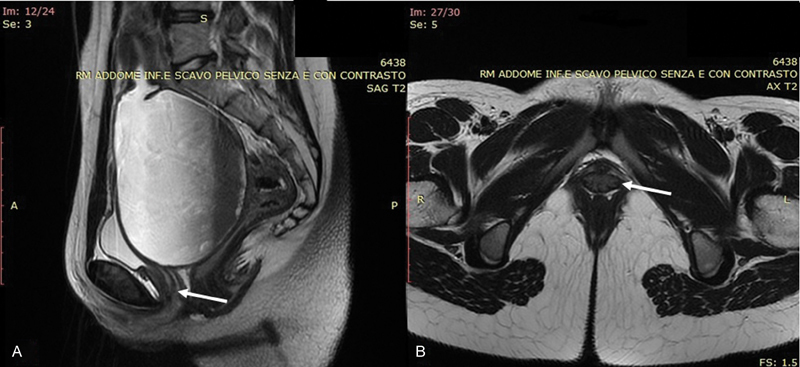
(A) Sagittal view of the magnetic resonance imaging scan: hematocolpometra and level of the septum (arrow); (B) transversal view of the septum (arrow).
